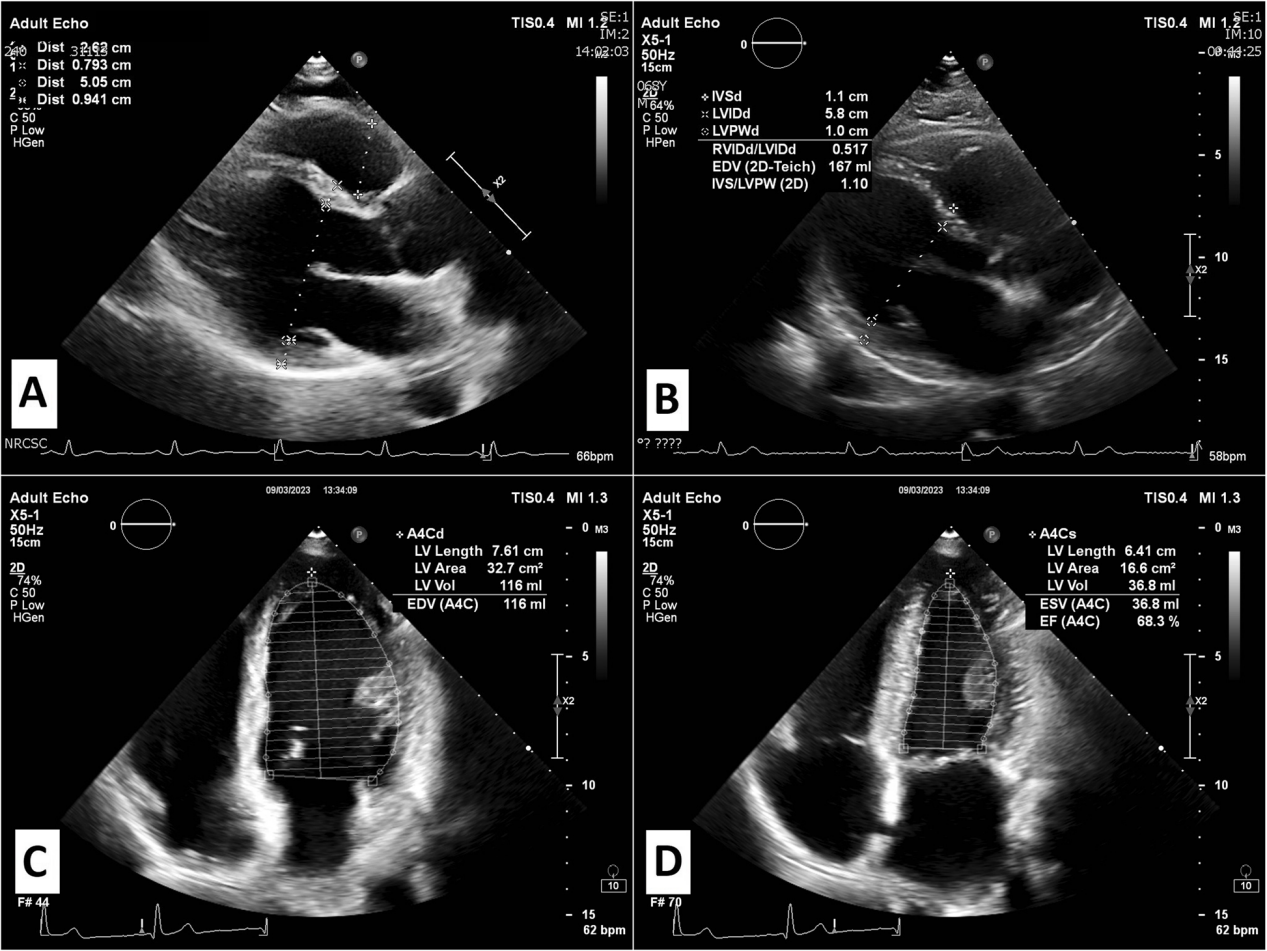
Representation of 2D echocardiography: A–B Parasternal long-axis view in diastole; C Apical 4-chamber view in diastole; D Apical 4-chamber view in systole. From these views, detailed assessment of left ventricular dimensions, wall thickness, and volumes can be performed
